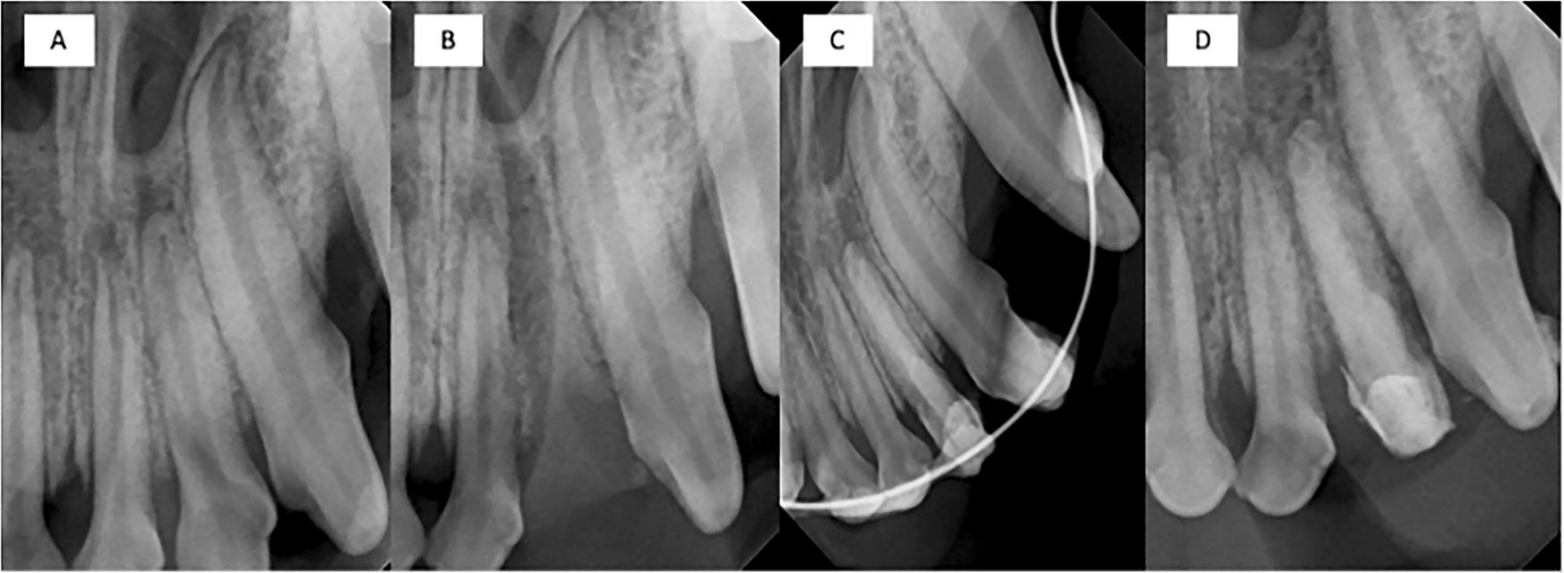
Radiographic images: figure includes radiographis for a maxillary left lateral incisor. A Day 0 pre-extraction. B Day 0 post-extraction. C Day post implantation. D Termination
